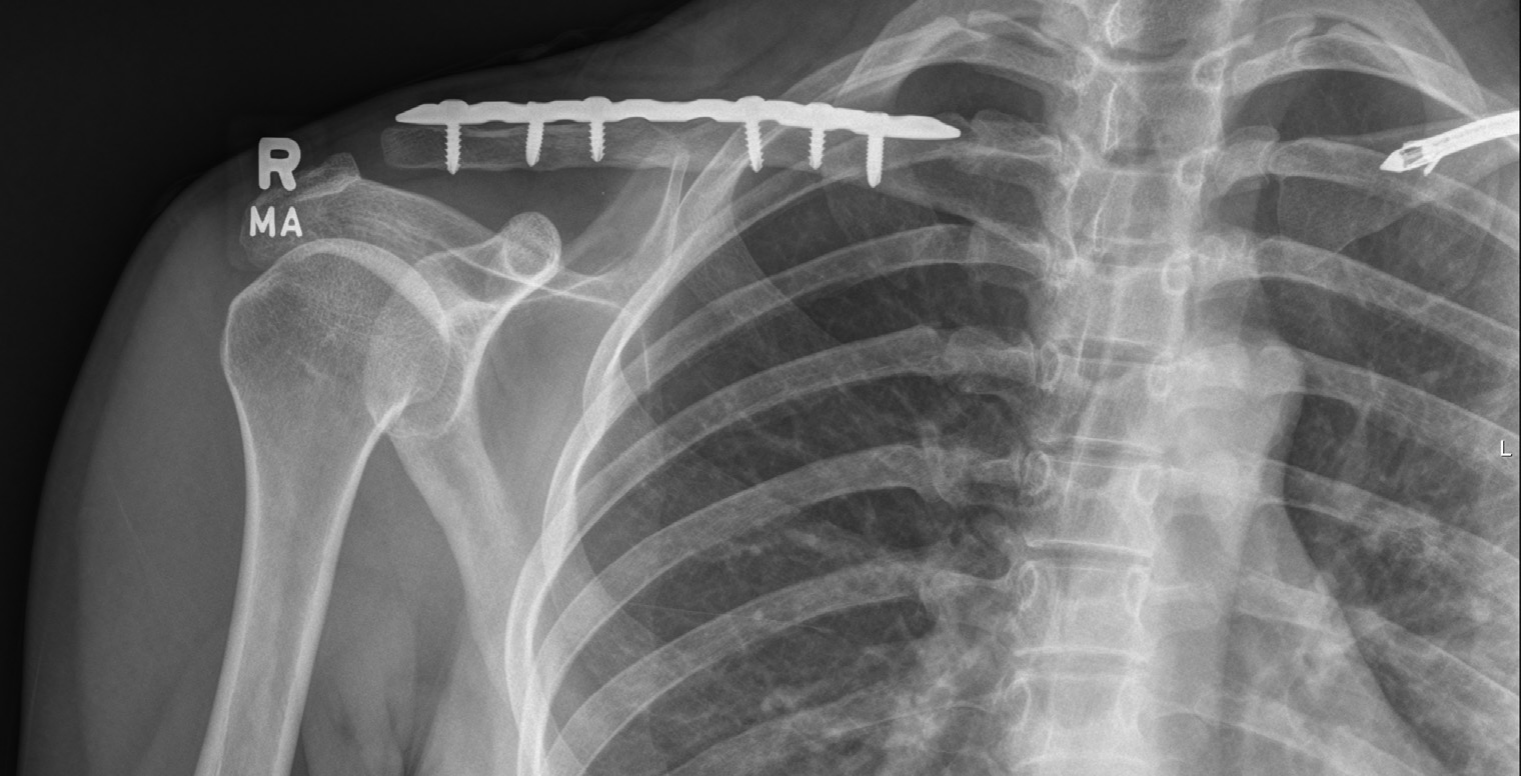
Axial radiograph of *Right* clavicle at 11 months postoperatively.
